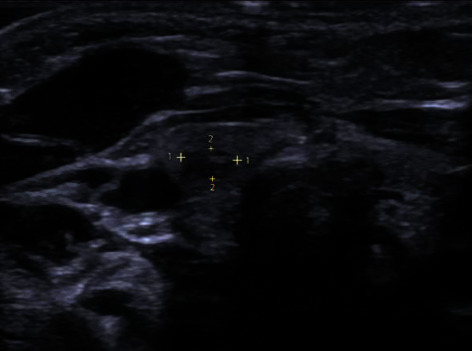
Ultrasound of the neck demonstrating 3.7 × 2.0 × 3.1 mm nodule in the right thyroid lobe.
